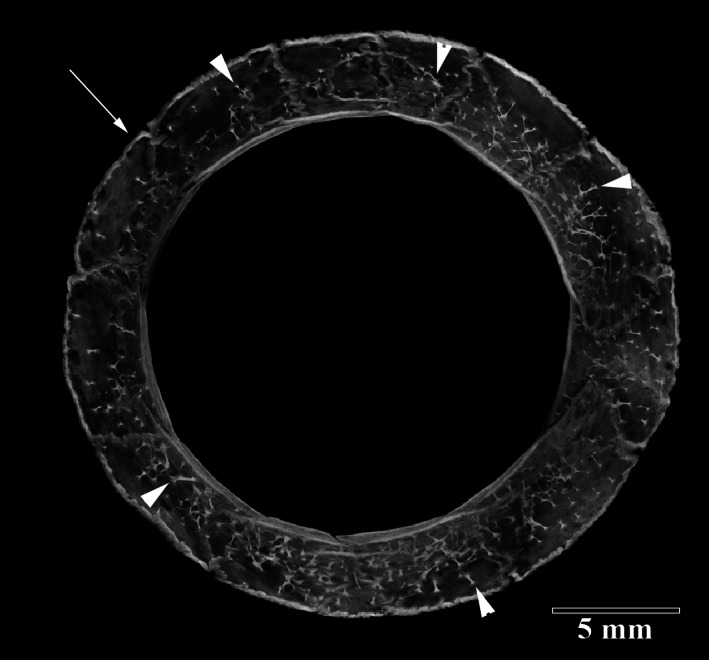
Micro‐CT maximum intensity projection image of the scleral ossicle ring of the right eye of a Snares crested penguin (*Eudyptes robustus*, AV 1178A). The interior of ossicles contained multiple internal struts (arrowheads) instead of solid bone. The external surface rim of the scleral ring consisted of more solid bone (arrow)
